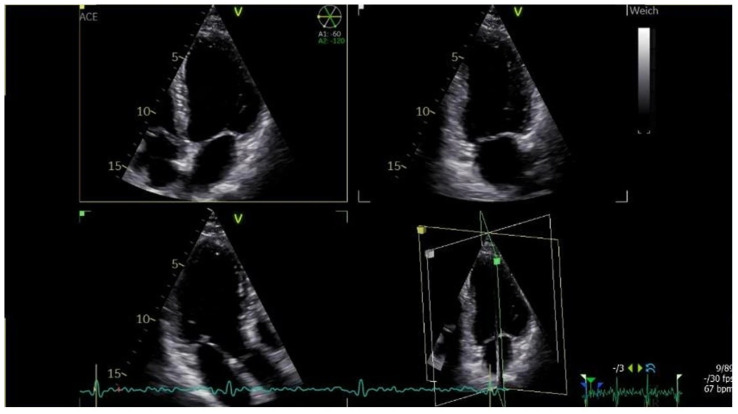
Day 64. Transthoracic echocardiography, triplane view demonstrating the apical four, two-, and three-chamber view. Demonstrating complete resolution of the left ventricular thrombus.
